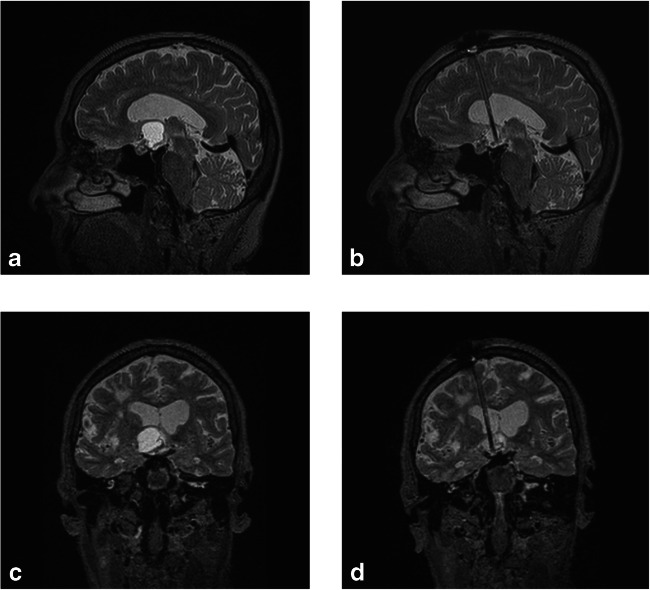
Preoperative sagittal (a) and coronal (c) T2-weighted MR images compared to postoperative sagittal (b) and coronal (d) T2-weighted MR images. Preoperative images (a, c) demonstrate the T2 hyperintense protein-rich craniopharyngioma cyst bulging above into the ventricular system. Postoperative images (b, d) demonstrate the volume reduction of the CSF-isointense cyst connected to the ventricular system via the implanted catheter
